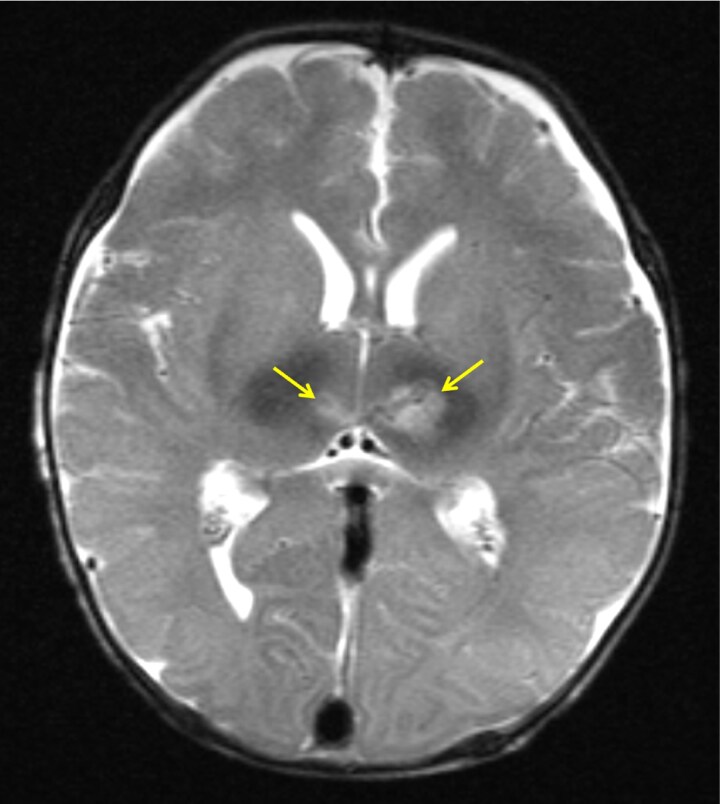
Axial T2 magnetic resonance imaging from hospital day 2 demonstrating bilateral thalamic hyperintensities (arrows).
